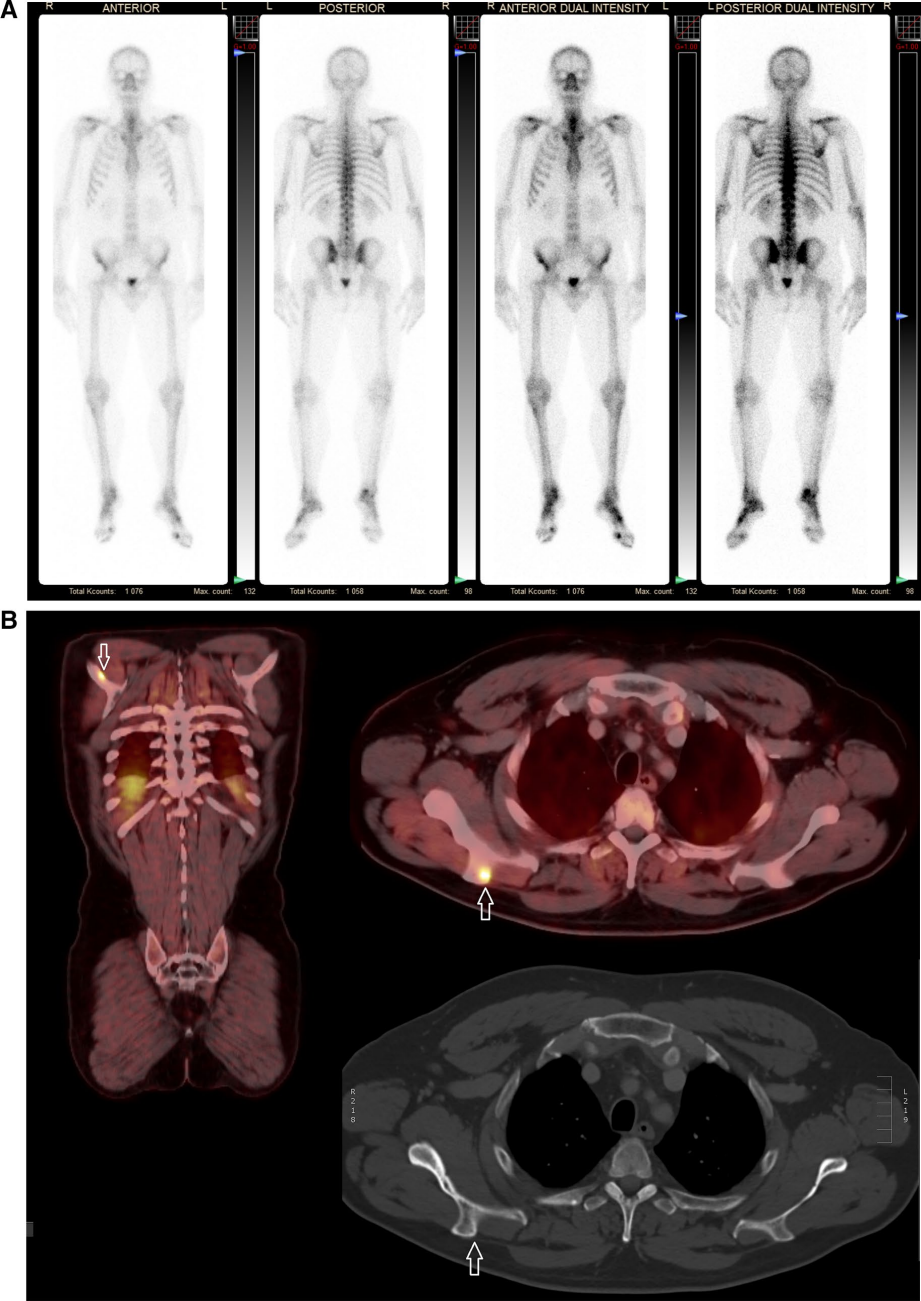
53-year-old man with a Gleason 4 + 4 PCa pT3 with PSA of 12.5 ng/ml. Primary staging with bone scan showed no lesions suspicious for bone metastases (a), but because he complained of right shoulder pain a ^18^F choline PET-CT was performed. This demonstrated focal tracer uptake in the spina scapulae (b, white arrow), indicating a bone metastase. On the corresponding CT image the scapula was unremarkable. Choline PET-CT may demonstrate bone metastases that are occult on bone scan and CT because it can detect changes in the bone marrow before osteoblastic reaction occurs. Nevertheless, bone scan currently remains the standard imaging modality in the primary staging of PCa
